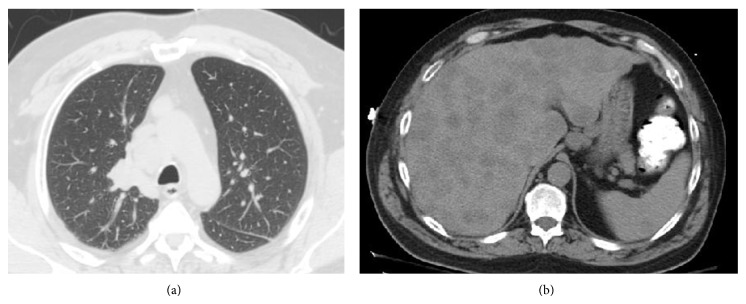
(a) CT of chest with contrast in mediastinal view displaying 2 cm right suprahilar soft tissue mass. (b) CT of abdomen showing multiple hepatic metastatic foci.
